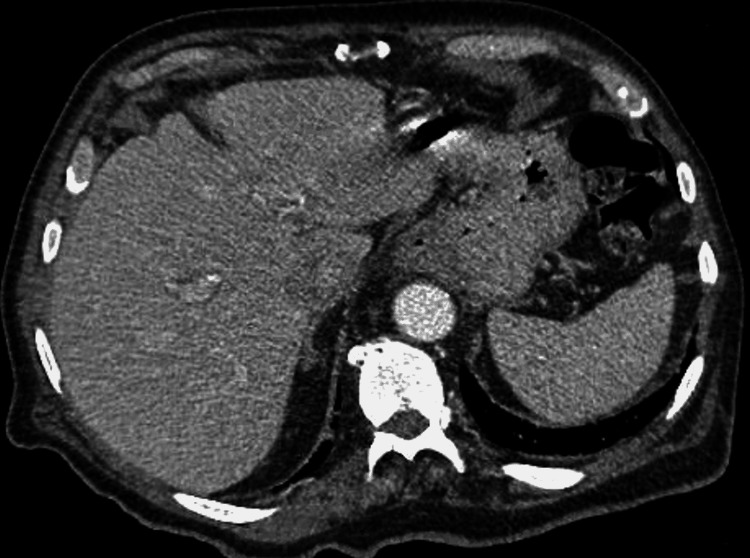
Computed tomography with intravenous contrast of the abdomen showing no acute pathology in the liver.
